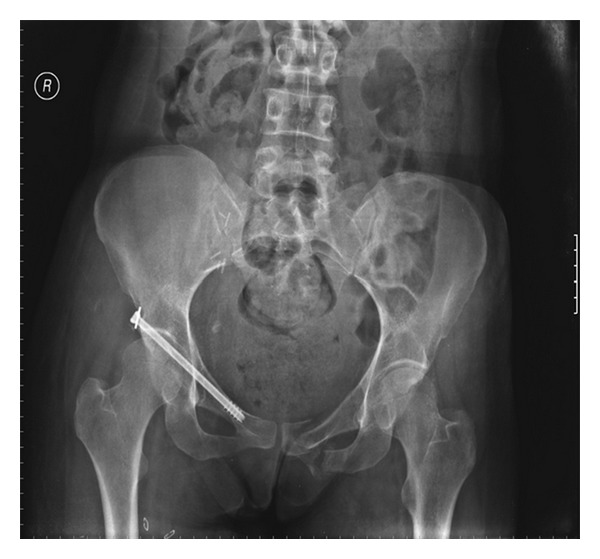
Postoperative X-ray: percutaneous fixation of anterior column fracture via 7,3 cannulated screw.
